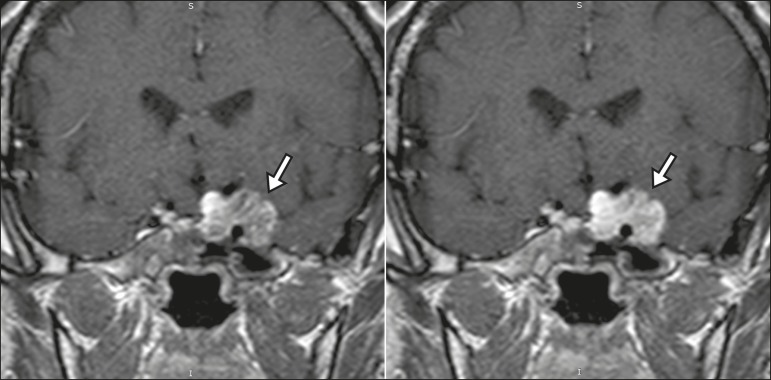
Hemangioma. Dynamic contrast-enhanced coronal T1-weighted MRI sequences
